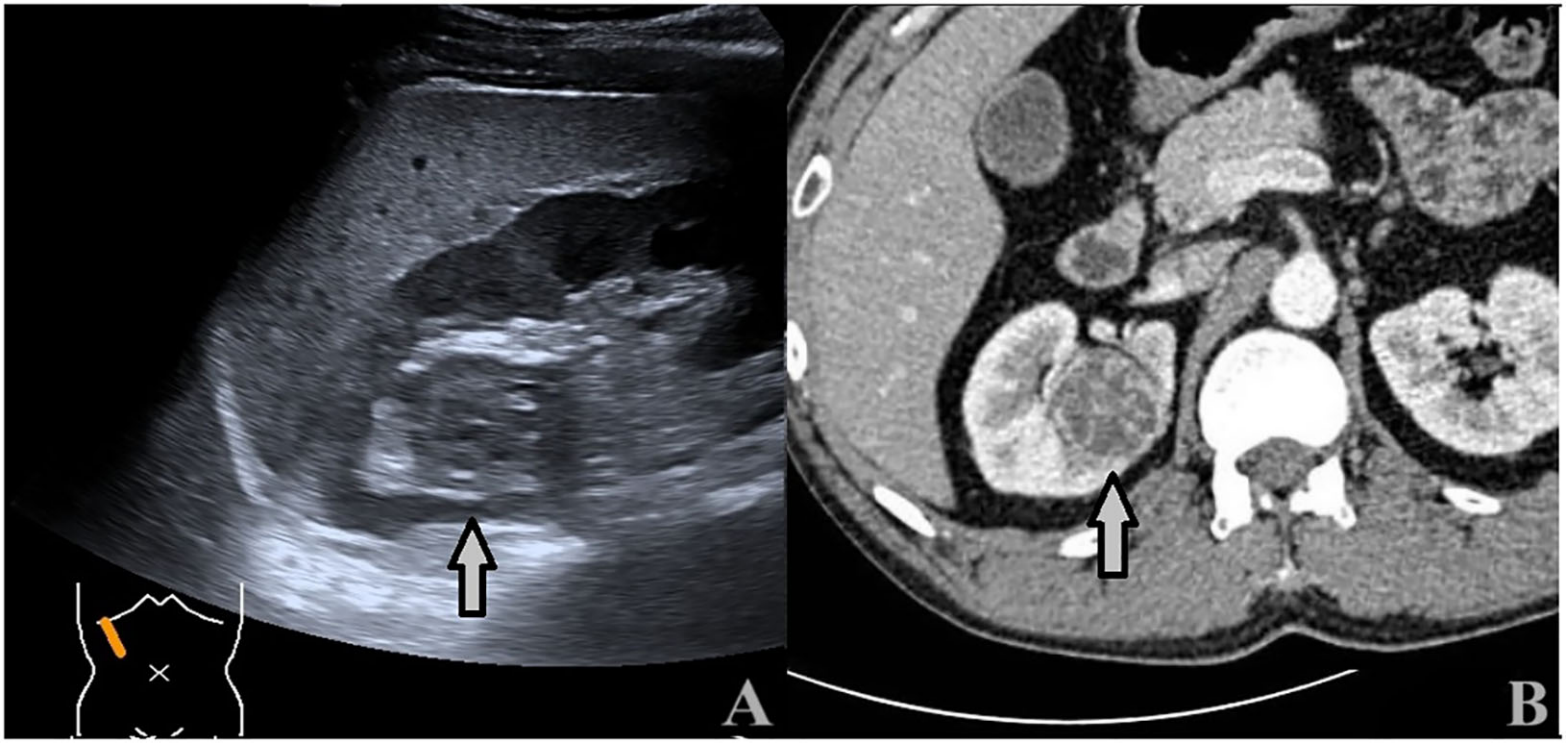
A 43-year-old man with a 3.5 × 3.4cm ccRCC. (A) Ultrasound showed a heterogeneous hypoechoic mass with intratumoral fluid areas located at the upper pole of the right kidney (arrows). (B) CECT: the tumor showed inhomogeneous hyperenhancement with many unenhanced areas (arrows).
